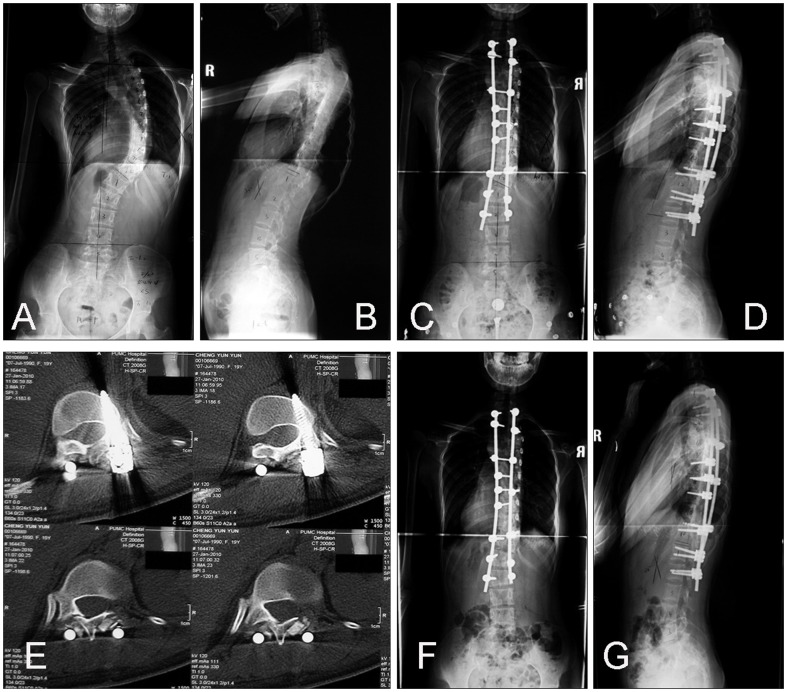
Patient is a 20 year-old male with congenital scoliosis. A and B, Standing preoperative anteroposterior and lateral radiographs. C and D, Standing anteroposterior and lateral radiographs 4 days after operation. E, Magnetic resonance images showing improper implant location. F and G, Standing anteroposterior and lateral radiographs 5 days after reoperation.
